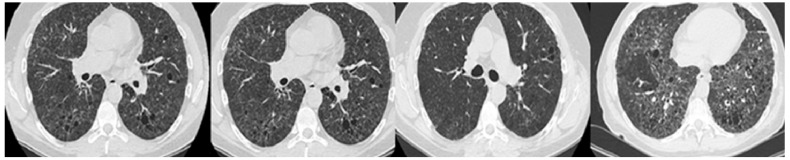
HRCT scans from 2018, 2019, 2020, and 2024, showing serial radiologic progression of chronic HP. The images demonstrate a gradual increase in mosaic attenuation, reticulation, and fibrotic changes, culminating in the development of honeycombing consistent with advanced pulmonary fibrosis.
